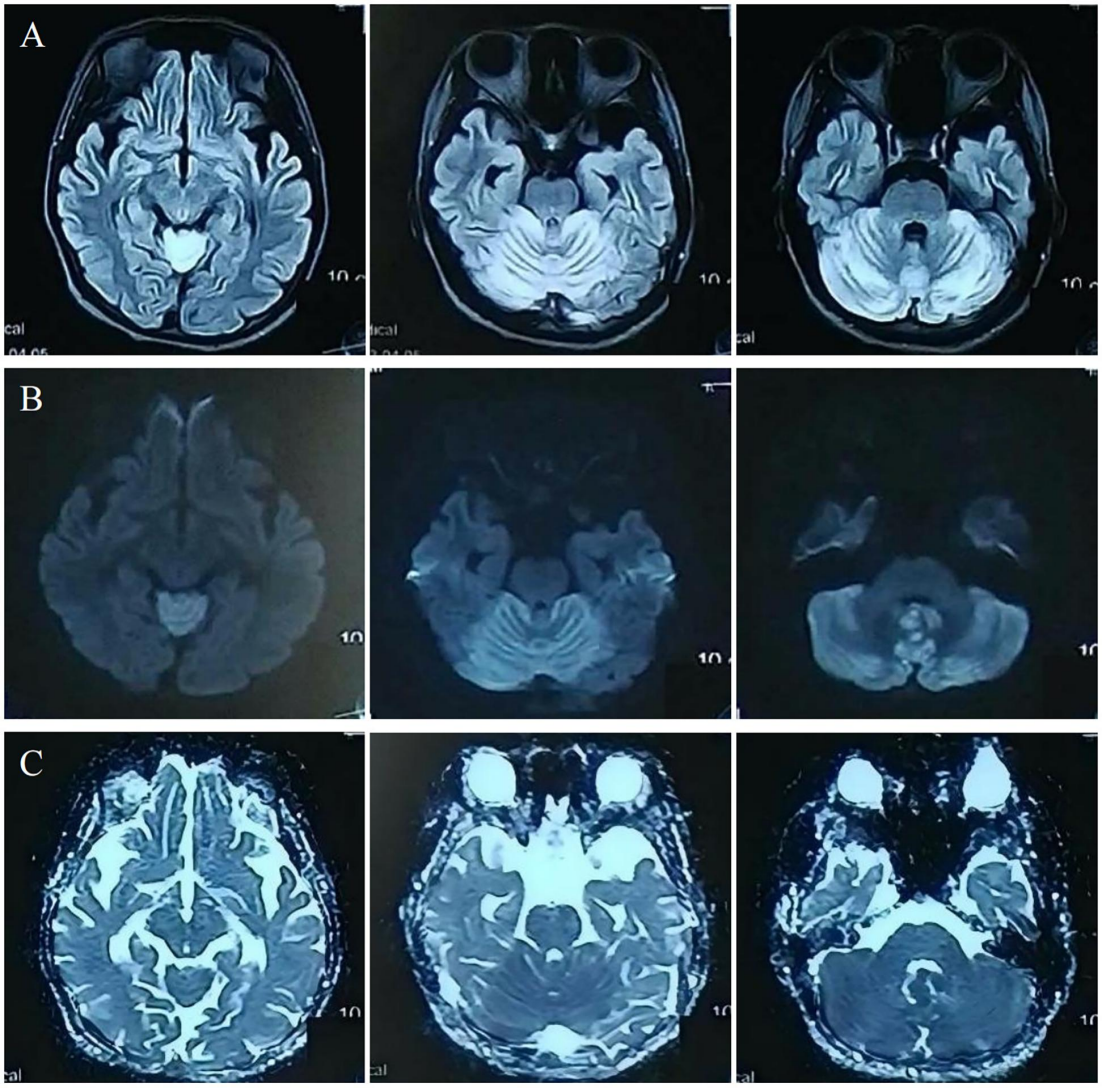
Acute MRI: cerebellar hyperintensities on FLAIR (A)/DWI (B); no ADC abnormality (C).
